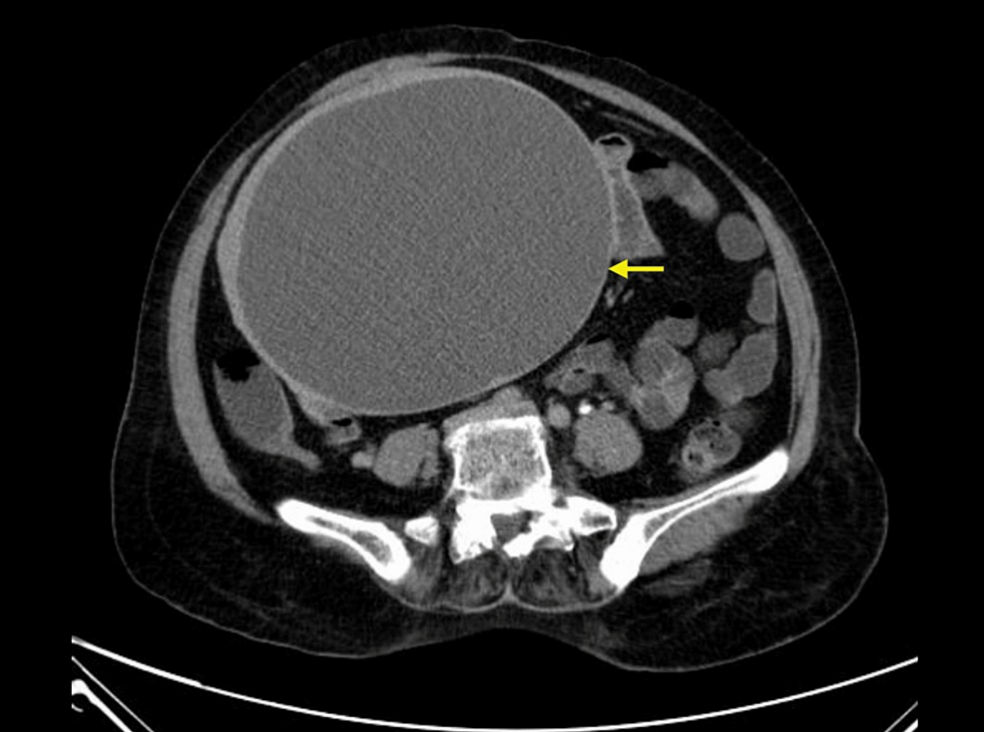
Contrast-enhanced computed tomography (CECT) of the abdomen showing a well-defined cystic lesion involving the right adnexa.
